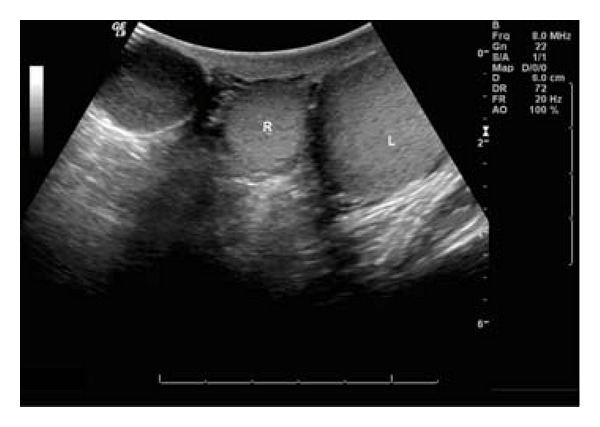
Coronal oblique sonogram shows an oval solid hypoechoic mass (arrow) separate from the right and left testes (T).
